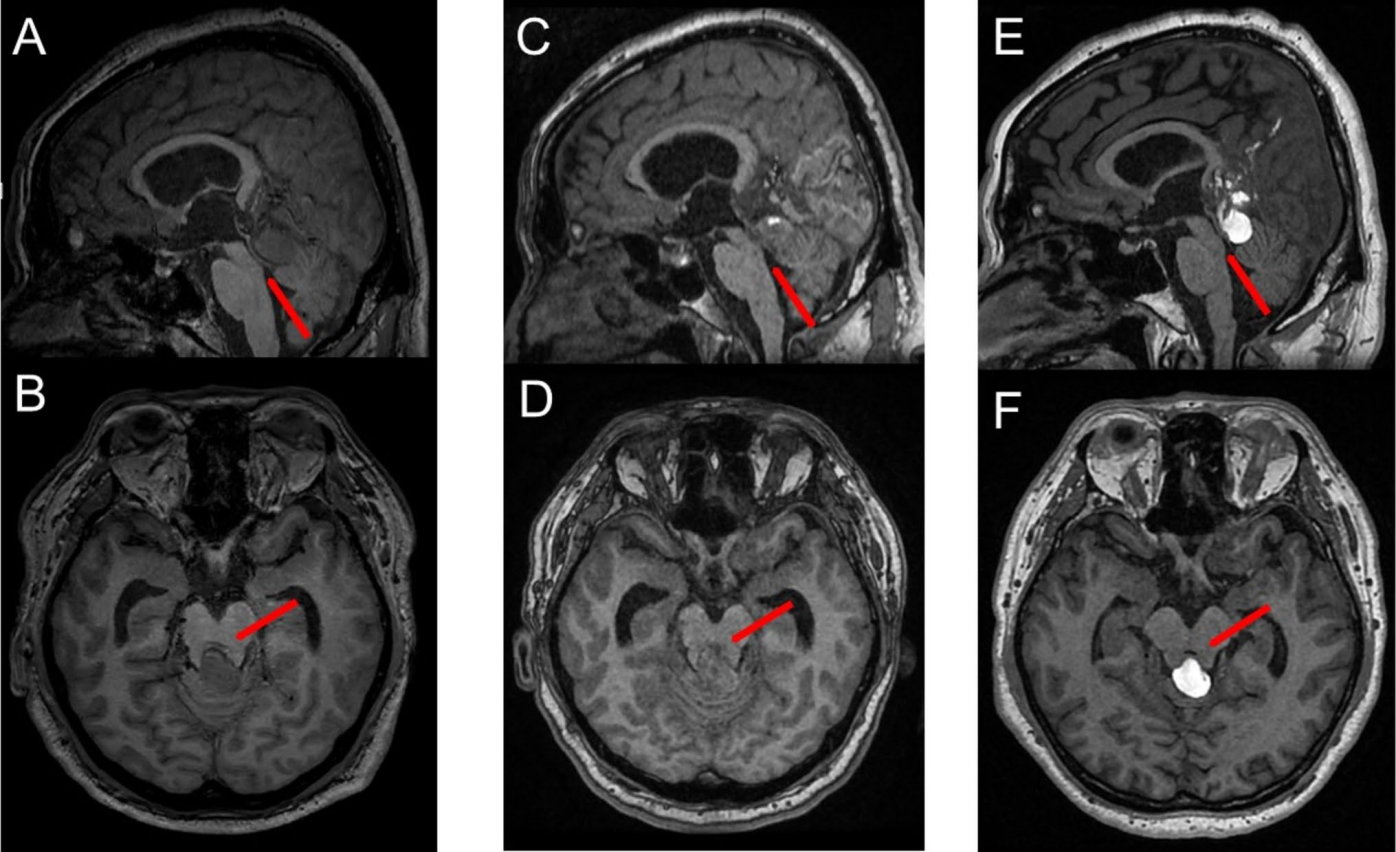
Initial brain magnetic resonance (MRI) showed a dilated varix of the dural AVF stenosed the aqueduct (red arrow) in the sagittal A and axial view B. Four days after the second TAE treatment of the dural AVF, disturbance of the consciousness worsened, hydrocephalus was worse on brain MRI A, showing the more dilated varix by the thrombus formation causing the occlusion of aqueduct (red arrow) in sagittal view C and axial view D. Six months after the TAE treatment, his brain MRI showed the shrinking of the dilated varix by the complete thrombus formation re-opening of the occluded aqueduct (red arrow) in sagittal view E and axial view F
